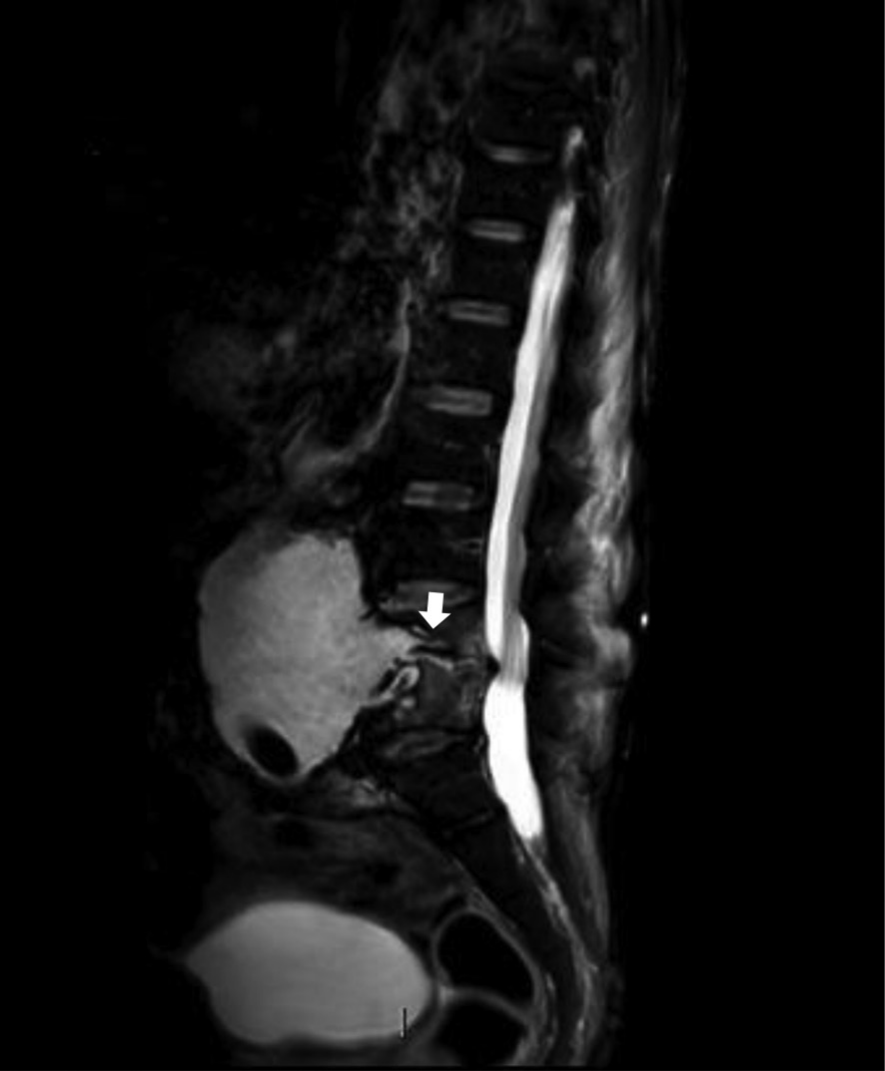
Sagittal MRI STIR showing communication of aneurysmal sac with L4/5 disc (arrow) with endplate destruction. STIR, short tau inversion-recovery.
